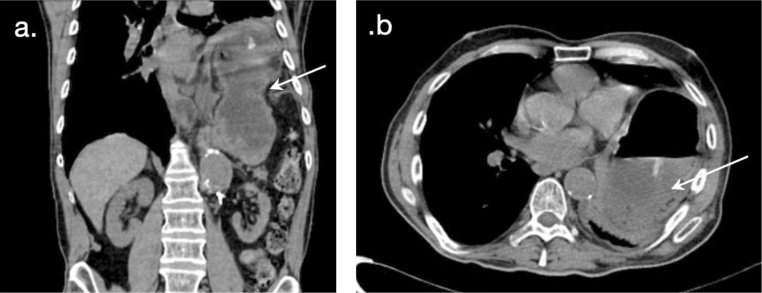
A–B. Pre-operative thoracoabdominal computed tomography scan: the seven-centimeter left hemi-diaphragmatic defect associated with herniation of the fundus, body and gastric antrum, along with the spleen.
